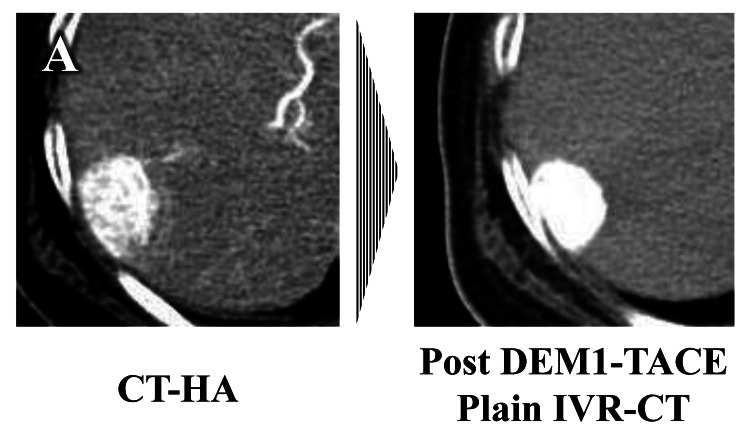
Typical pattern of homogeneous contrast medium retention evaluated by IVR-CT in target nodules after DEM1-TACE CT-HA: computed tomography-during hepatic arteriography; DEM1-TACE: drug-eluting bead (DEB)-TACE using DC Bead M1^TM^; IVR-CT: interventional radiology-computed tomography (A) Homogeneous contrast medium retention appeared in IVR-CT after DEM1-TACE
